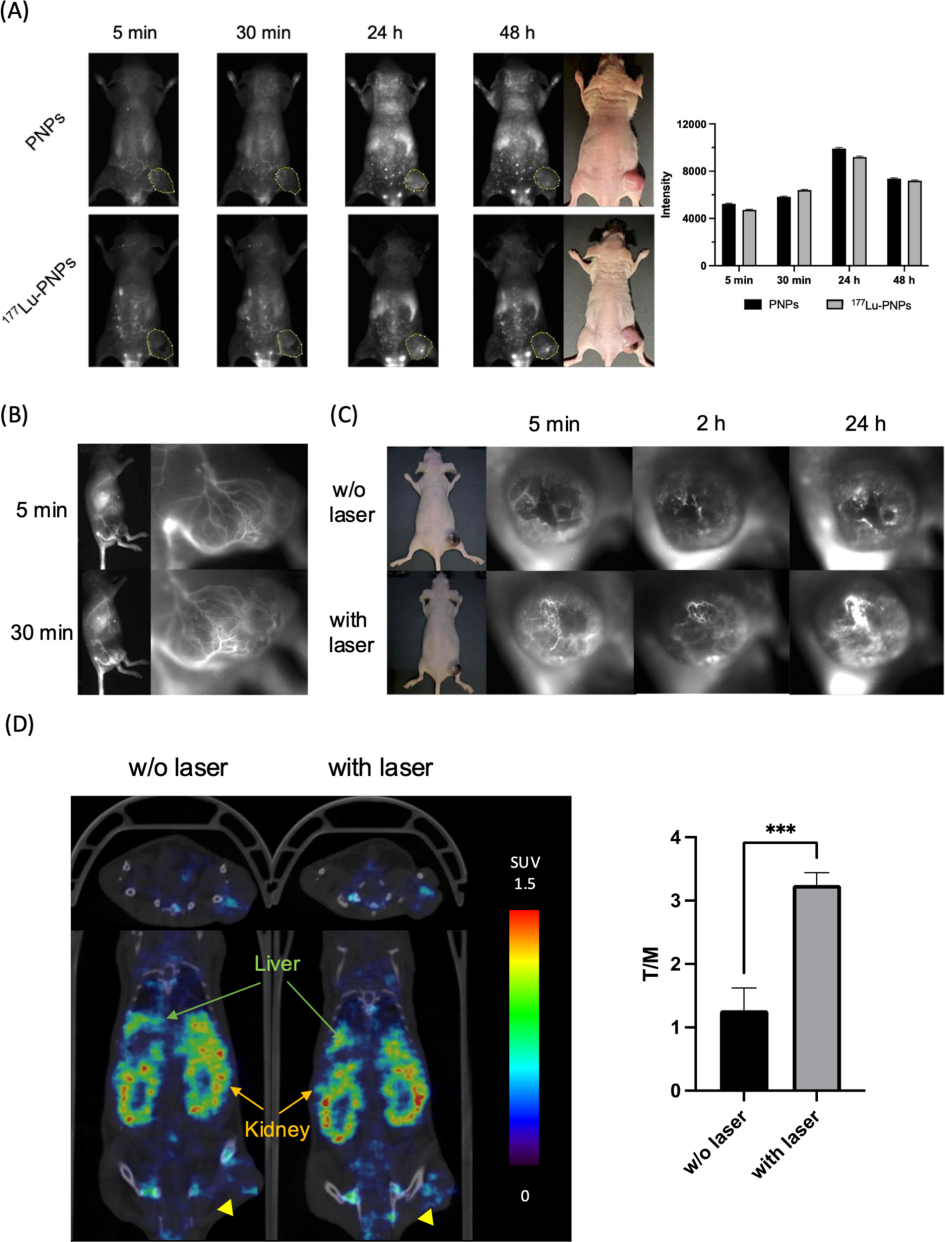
NIR-II imaging and SPECT
